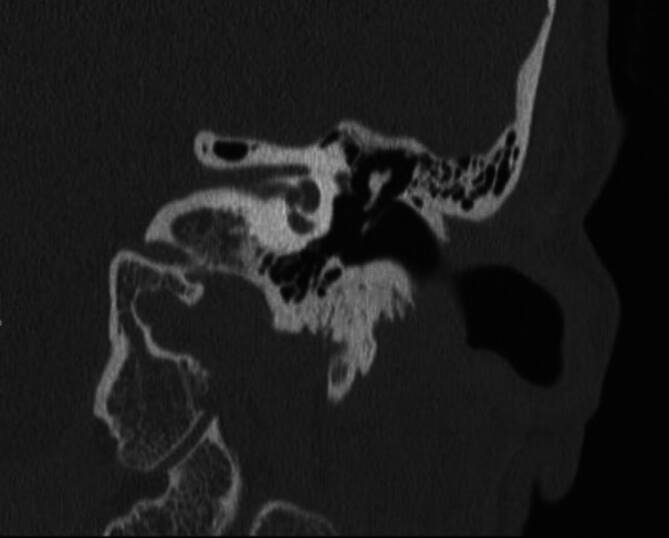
Koronare Darstellung der Gehörknöchelchenkette in der Felsenbein-CT
